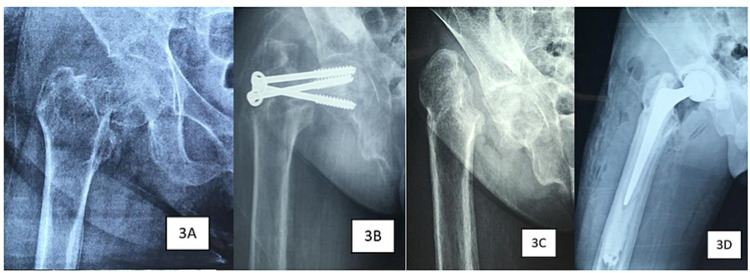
Right hip plain radiographs of the patient in Case 2 show intracapsular neck of femur fracture immediately after trauma (3A), failure of cancellous screw fixation two months post-operation (3B), post-parathyroidectomy (3C) and post-total hip replacement (3D).
